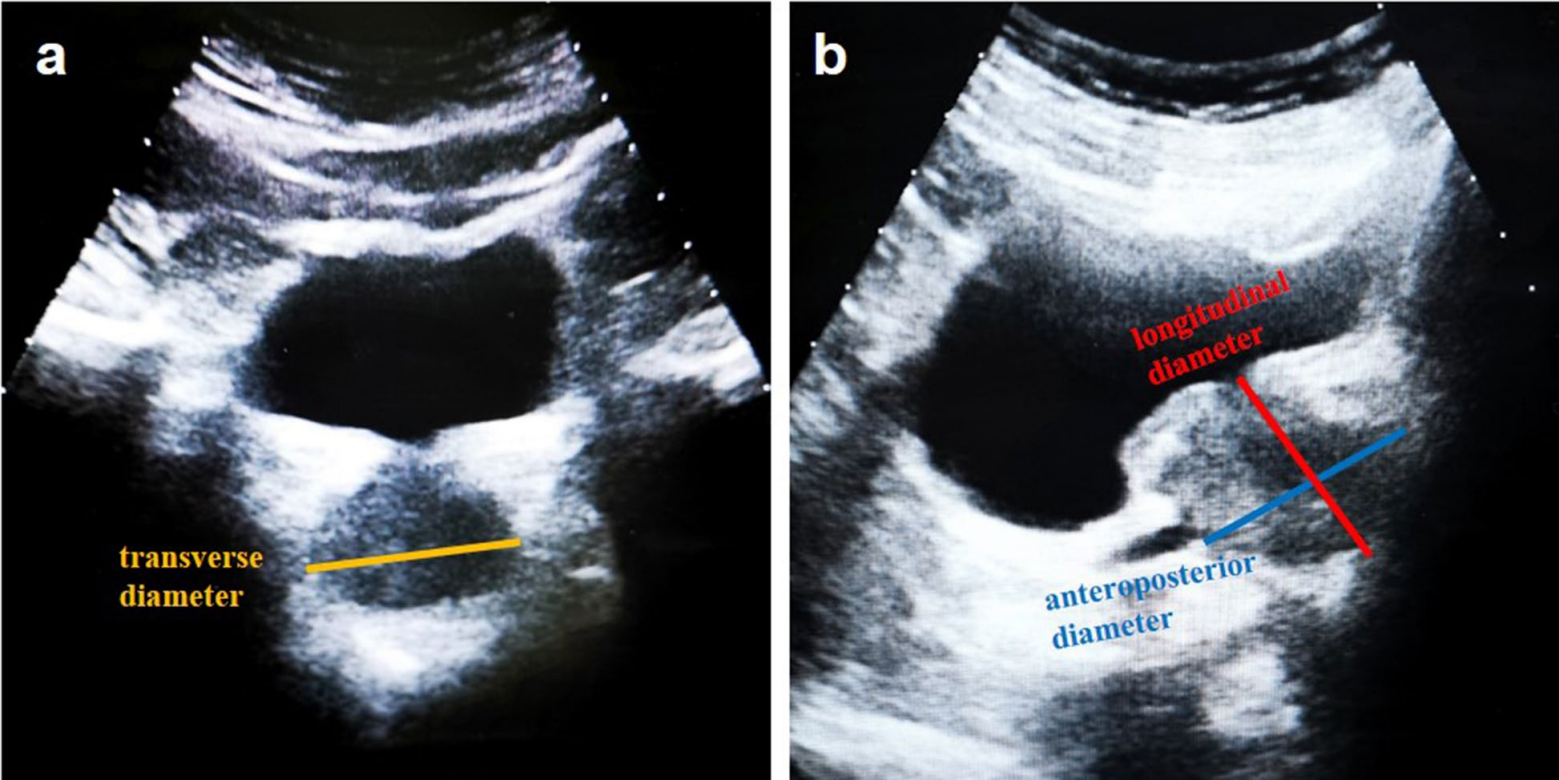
Measurement of prostate diameter when using ellipsoid formula to calculate prostate volume on transabdominal ultrasound. (a) maximum transverse diameter (width) measured on axial scanning. (b) Maximum longitudinal diameter (length) and maximum anteroposterior diameter (height) measured on midsagittal scanning
